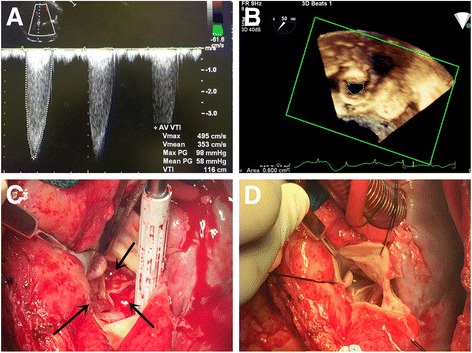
a, b The TEE detected an increased mean transvalvular gradient of 58 mmHg, a peak velocity of 4.95 m/sec and a restricted bioprosthesis opening of about 0.6 cm^2^. c The intraoperative view of the bioprosthetic valve, with massive thrombus (*in black arrow*) involving all the leaflets. d The bioprosthetic valve after thrombectomy showed smooth surface and normal function. (*TEE* transesophageal echocardiography)
